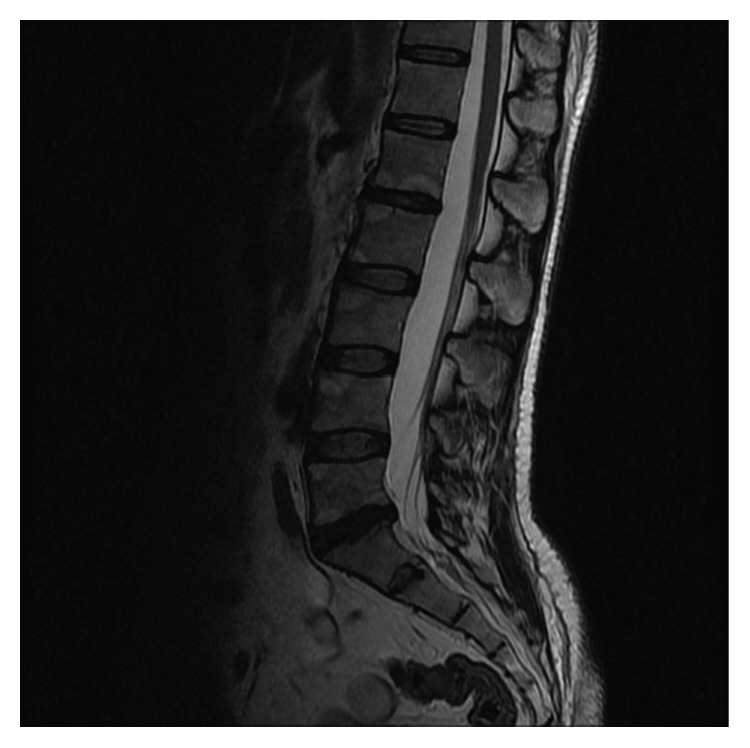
T2 sagittal MRI scan of the lumbar spine revealing Modic type 2 degeneration.
